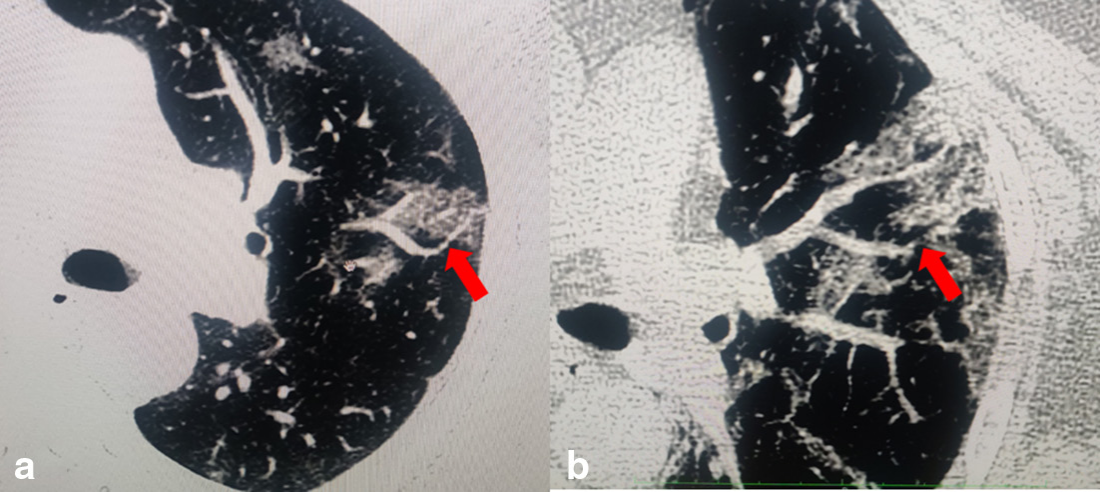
Axial chest CT images in lung window settings obtained through the upper
